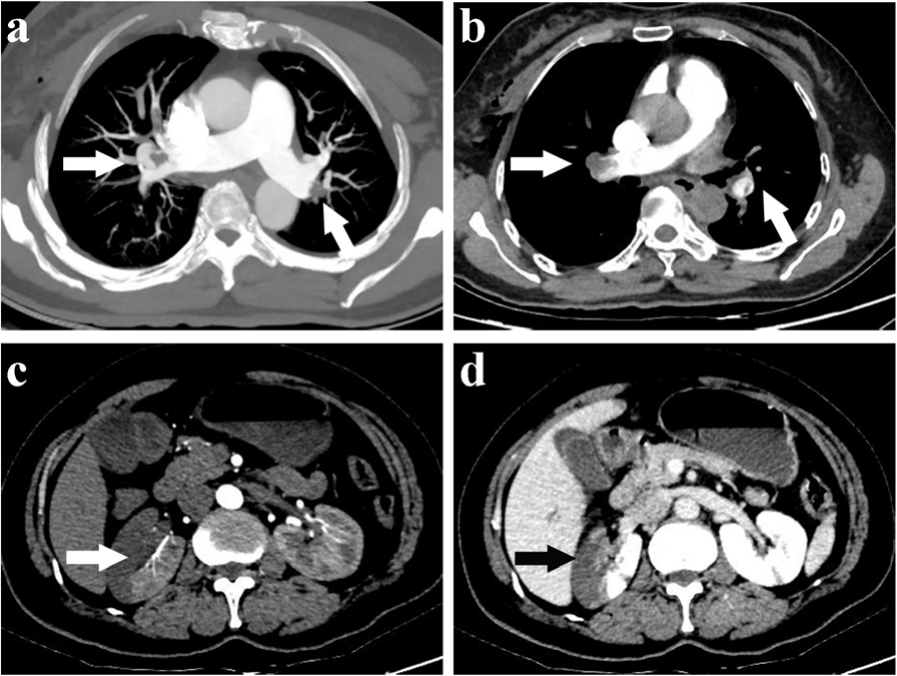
Contrast-enhanced CT angiography demonstrated a filling defect of main bilateral branches of pulmonary trunk (a and b), and an absent enhancement of a segment of right renal parenchyma in the upper pole (c and d). Ill-defined border and perinephric stranding suggested renal artery embolism was acute
